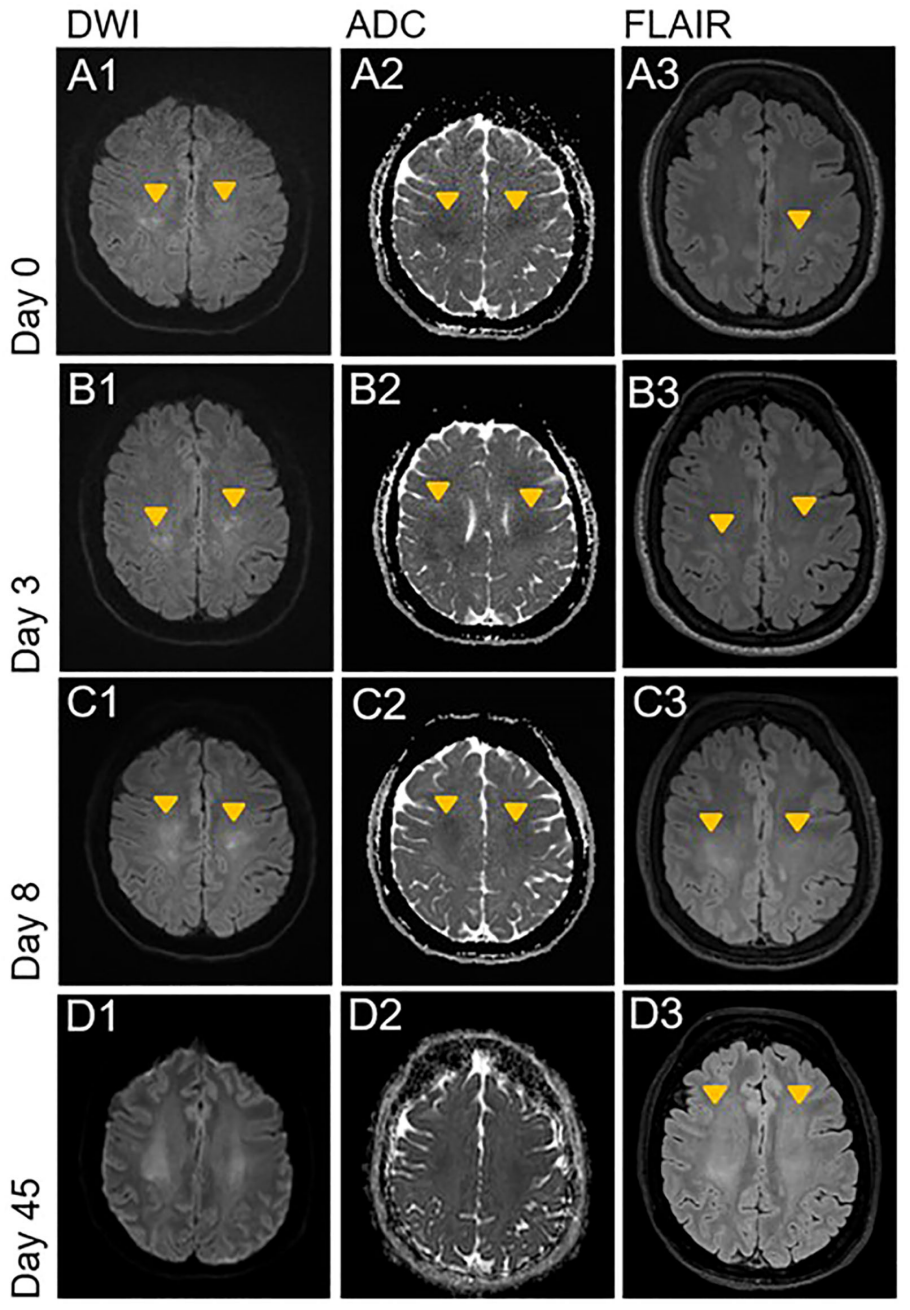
Acute progressive leukoencephalopathy shortly after initiation of therapy with daratumumab. DWI, ADC and FLAIR sequences of the initial brain MRI examination on day 0 and follow up imaging on day 3, 8 and 45. Orange arrowheads indicate new or progressive lesions. (A1-A3) Bilateral, diffuse diffusion restriction in the deep white matter of the parietal centrum semiovale with discrete FLAIR hyperintense correlate on left parietal side. (B1-C3) Progression of the bilateral and diffuse diffusion restriction with T2w and FLAIR hyperintense correlate on the left parietal side. New correlating FLAIR hyperintensities on the right parietal side. Start of pseudo-normalization of ADC in the more parietal areas (C2). (D1-D3) Further progression of the bilateral confluent FLAIR hyperintensities to the frontal white matter of centrum semiovale. Almost complete pseudonormalization of ADC with residual diffusion restriction. FLAIR, Fluid attenuated inversion recovery; DWI, Diffusion weighted imaging; ADC, Apparent diffusion coefficient.
